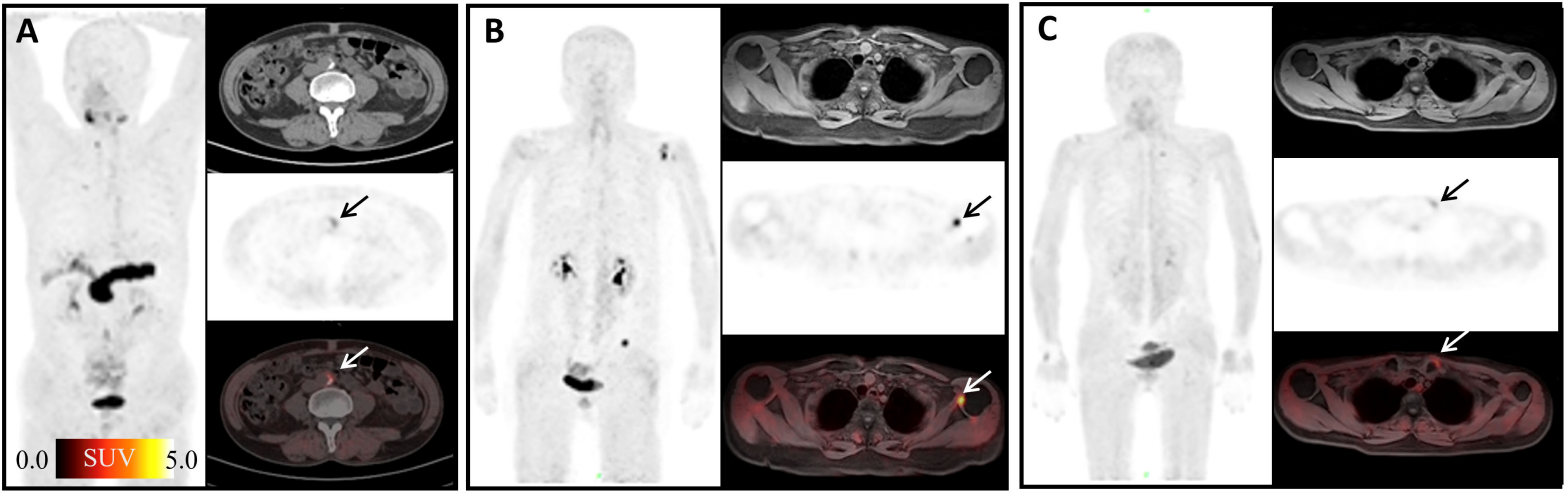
Physiological uptake of ^68^Ga-FAPI-04. (A) A 72-year-old man with duodenal papillary tumor; arrows show atherosclerosis of the abdominal aorta with SUV_max_ =4.65. (B) A 70-year-old woman presented with adenocarcinoma at the descending colon–sigmoid junction; arrows show left shoulder arthritis with SUV_max_ =11.09. (C) A 55-year-old woman with signet ring cell carcinoma of stomach 2 months after ESD; arrows show left sternoclavicular arthritis with SUV_max_ = 8.21.
